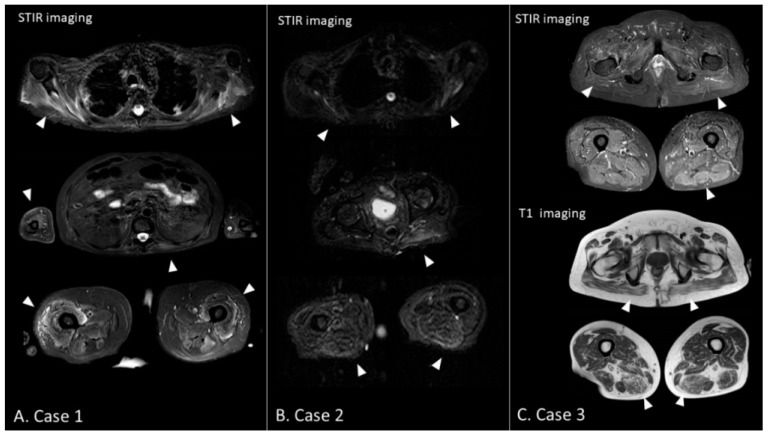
Muscle MRI findings. (A) Case 1: Hyperintense lesions are observed in the shoulder girdle muscles, biceps brachii, left paraspinal muscles, and anterior compartment of the thighs in STIR imaging (triangles). (B) Case 2: Hyperintense lesions are observed in the shoulder girdle muscles, left gluteus maximus, and posterior compartment of the thighs in STIR imaging (triangles). Furthermore, marked atrophy is seen in the thighs (triangles). (C) Case 3: In STIR imaging, hyperintense lesions are observed in the limb-girdle muscles, including the gluteus maximus and posterior compartment of the left thigh. In T1 imaging, hyperintense lesions are more prominent than in the STIR imaging, suggesting fatty infiltration, a finding of chronic phase ICUAW (triangles). STIR, short T1 inversion recovery.
